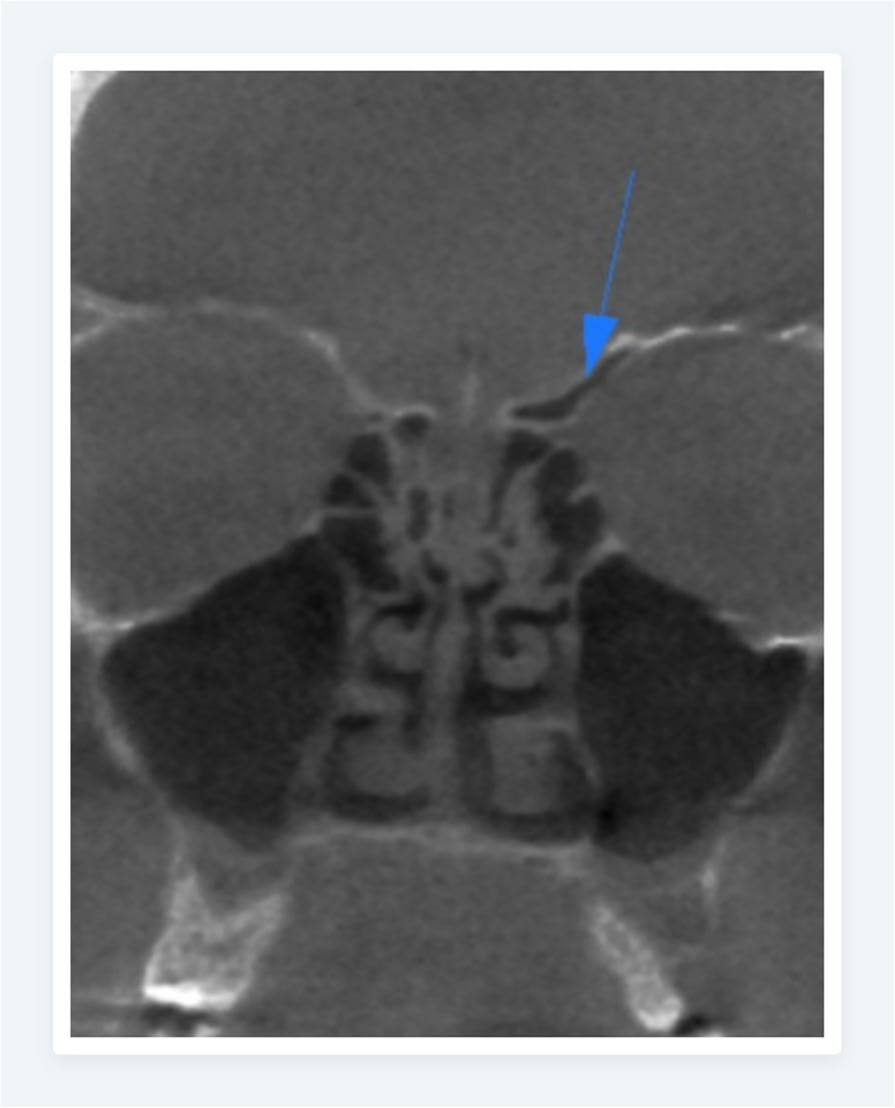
Coronal CBCT scan of the left supraorbital ethmoid cell
